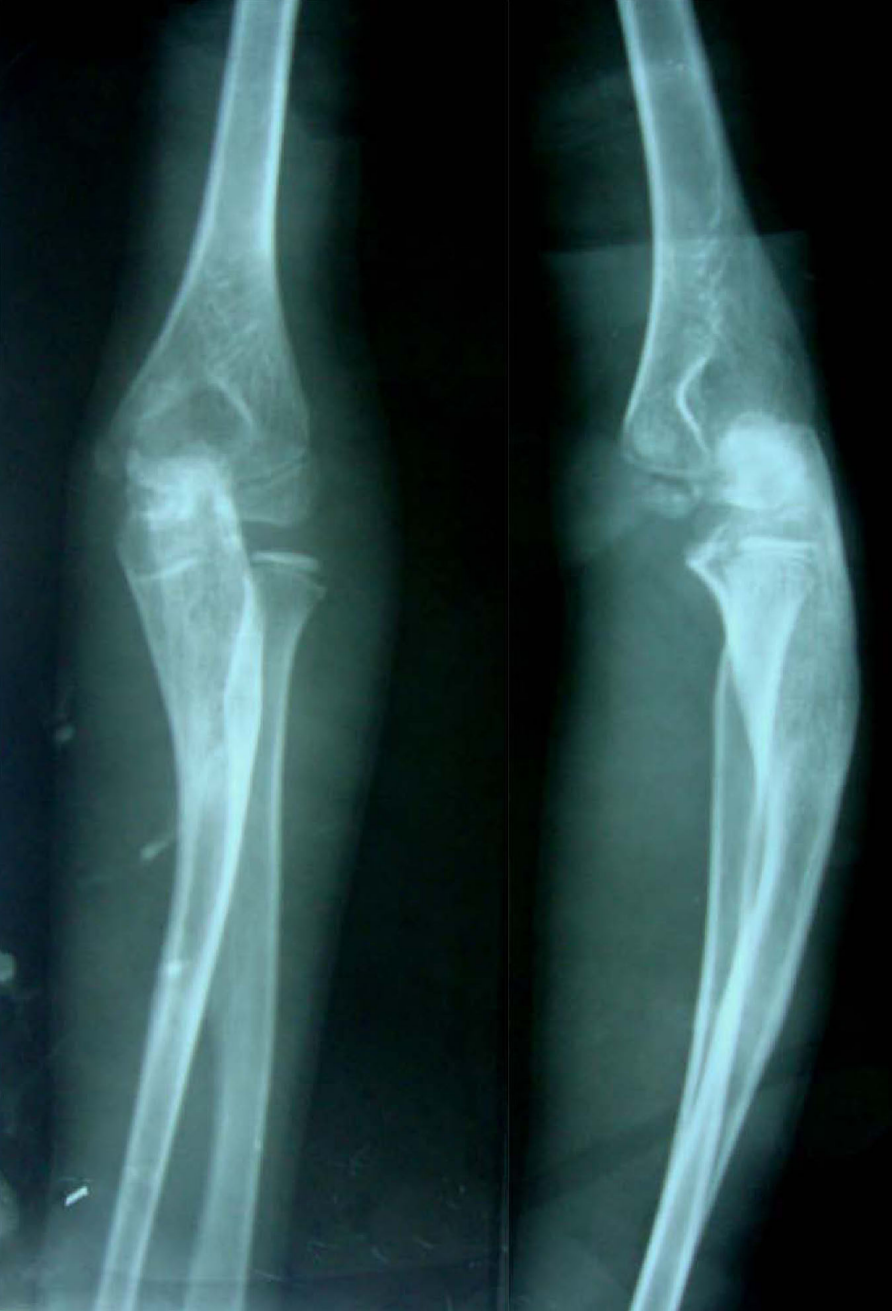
Radiograph taken at the final follow-up visit showing relocation of the radial head (Patient 2).
